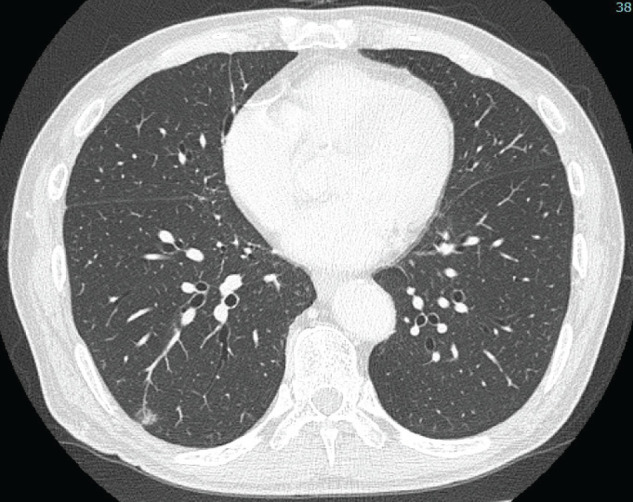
Chest CT showing a 9-mm pure GGN in segments 8/9 (S8/9) of the right lower lobe (Case 2). GGN, ground-glass nodule
